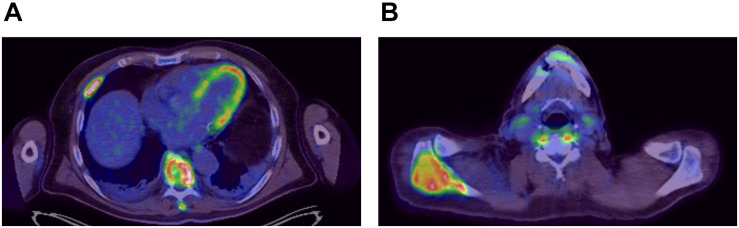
FDG-Positron emission imaging at first relapse of ALCL showing hypermetabolic lesions around the spine (A) and within the right scapula (B).
